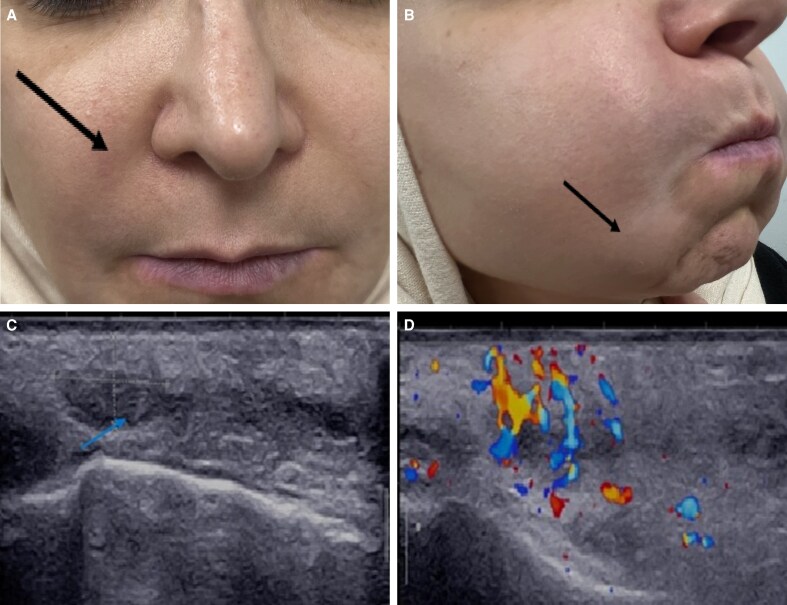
(A, B) A 52-year-old female with a history of PCL injection in the bilateral nasolabial folds and marionette lines (black arrows). (C, D) The ultrasound axial view reveals multiple hypoechoic nodules containing echogenic foci (blue arrows) and inner vascularity, which are compatible with granulomas.
